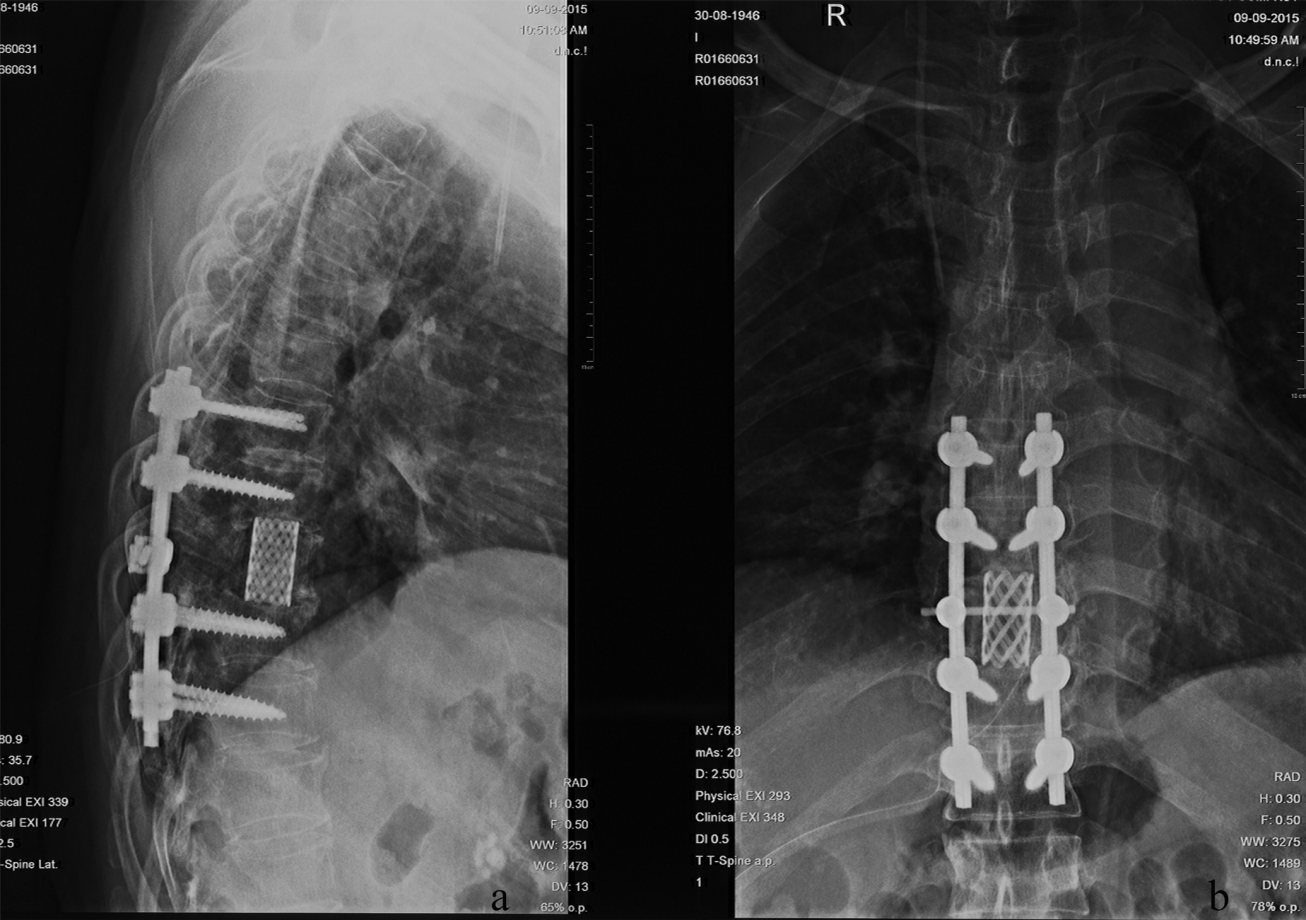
A.-B. Postoperative X-ray outcome at the final follow-up 59.0 months after surgeries and adjuvant therapies.
